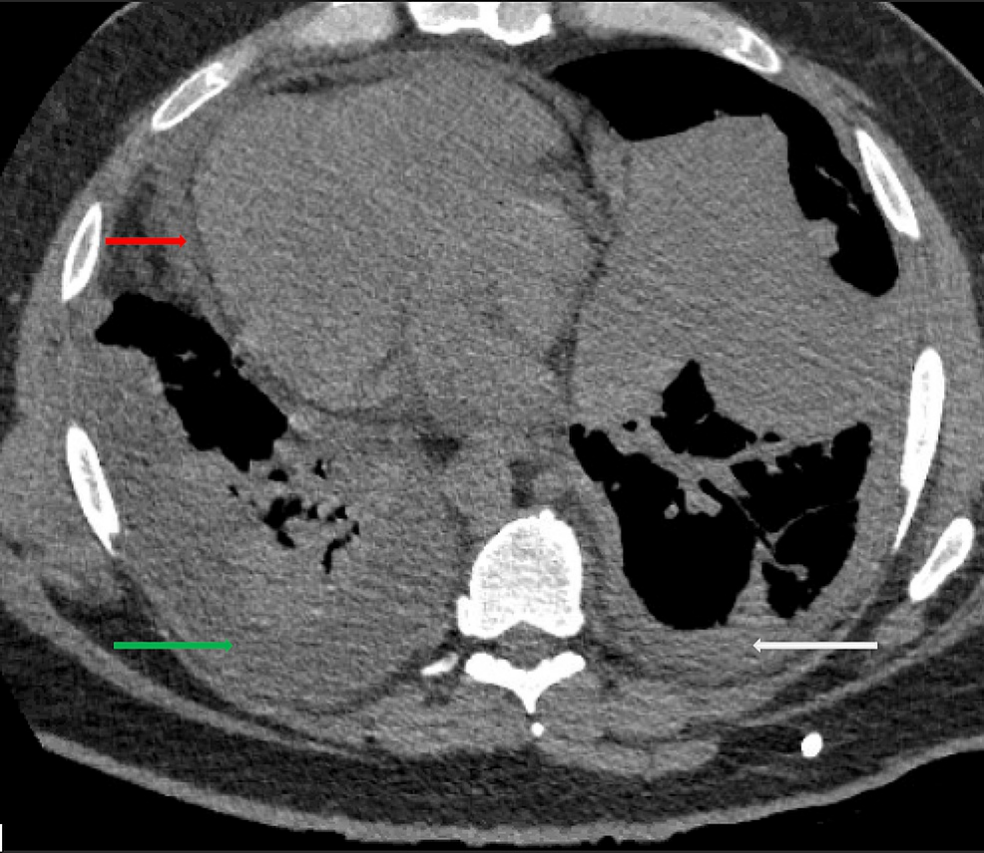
CT chest showing moderate pericardial effusion (red arrow), moderate right-sided pleural effusion (green arrow), and mild left-sided pleural effusion (white arrow)
